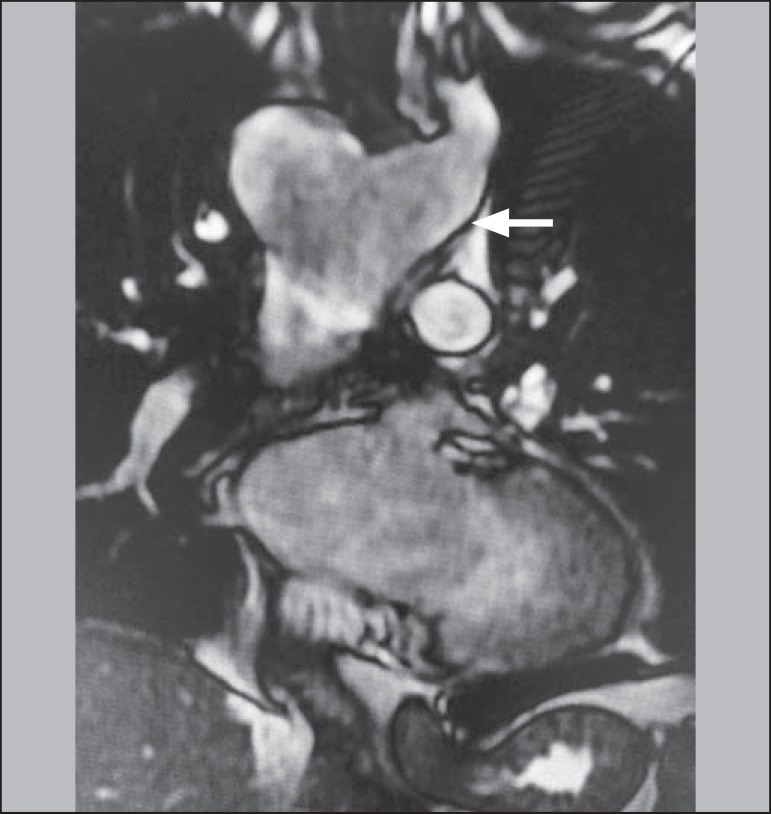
Coronal T2-weighted spin-echo magnetic resonance imaging showing Kommerell's
